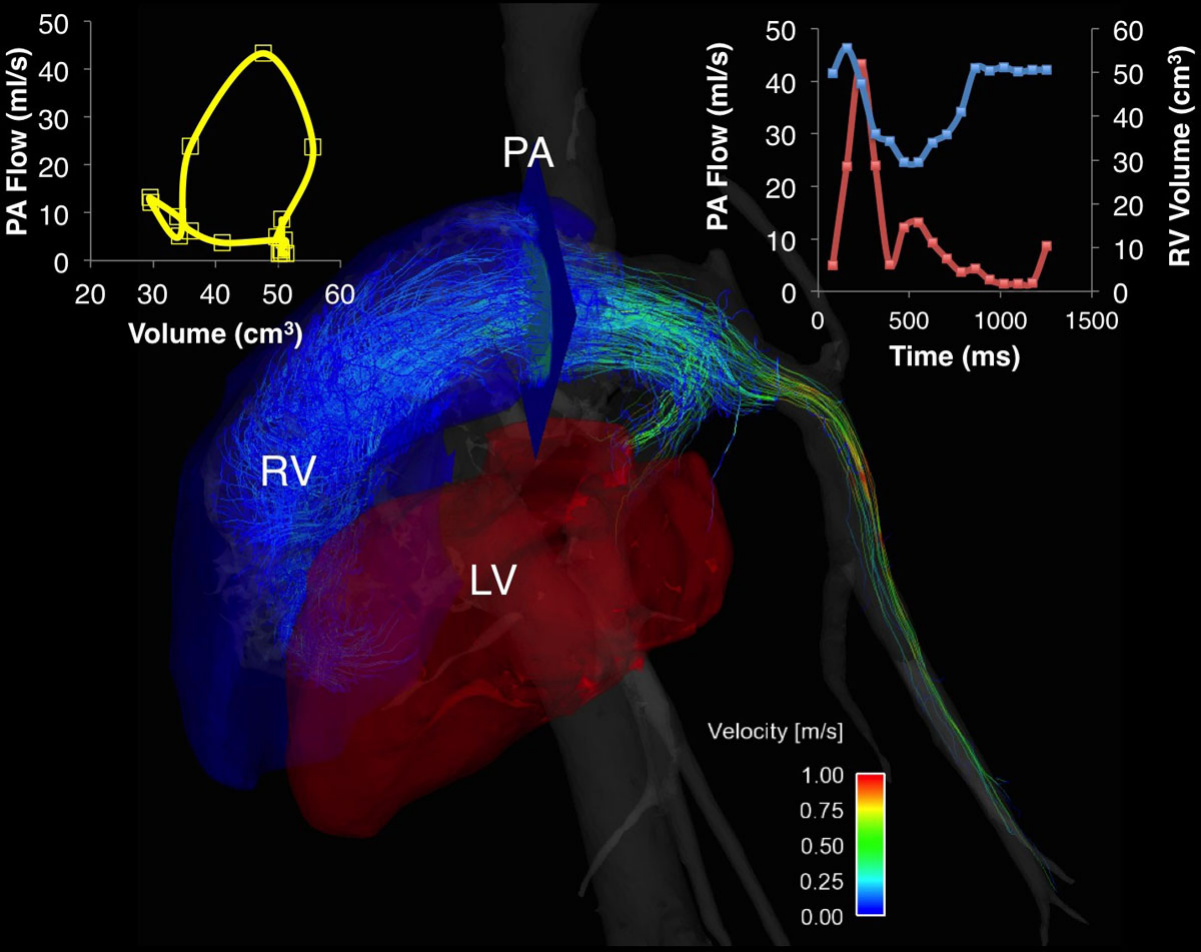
Visualization and quantification of pulmonary arterial flow and right ventricular volume using 4D Flow MR images.
